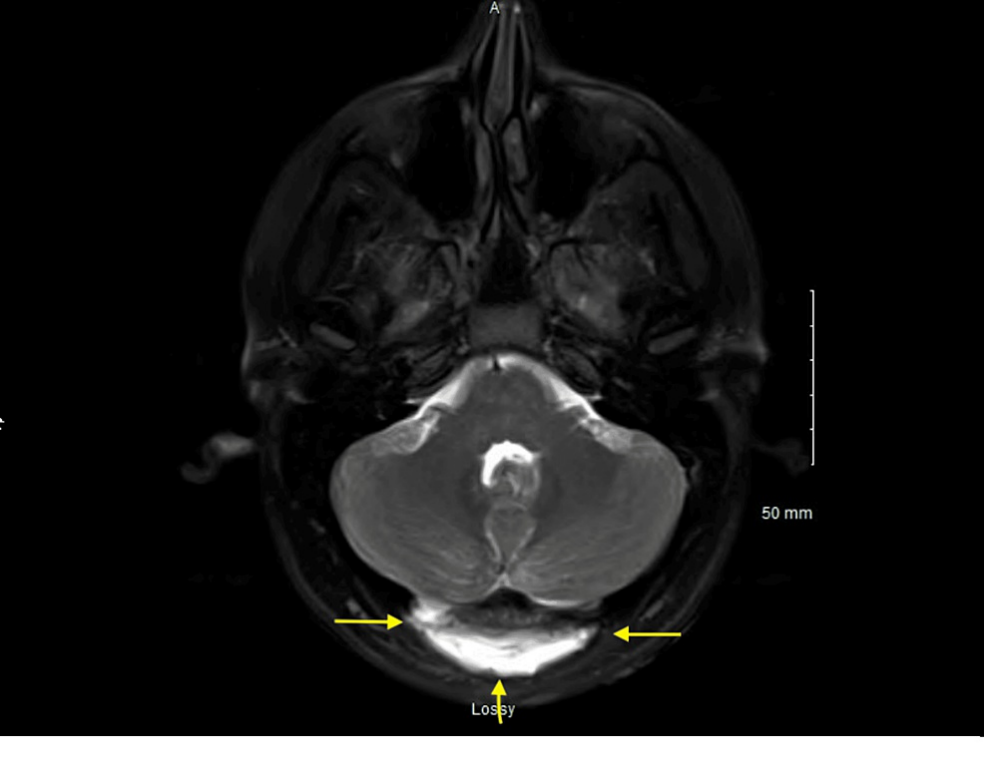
MRI performed on the patient’s initial arrival to the emergency room, demonstrating a complex fluid collection in the dorsal soft tissues measuring 1 x 6 x 7.5 cm.
